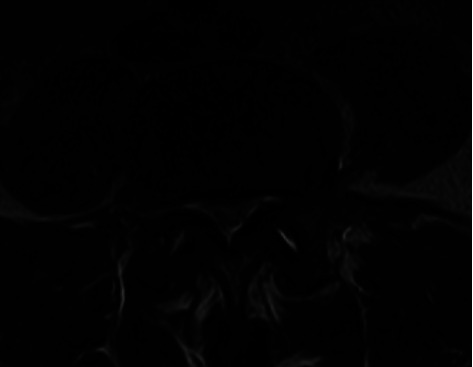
Axial T2-weighted image at the L4–L5 level demonstrates enlarged facet joints bilaterally with marginal osteophytes and joint effusions.
